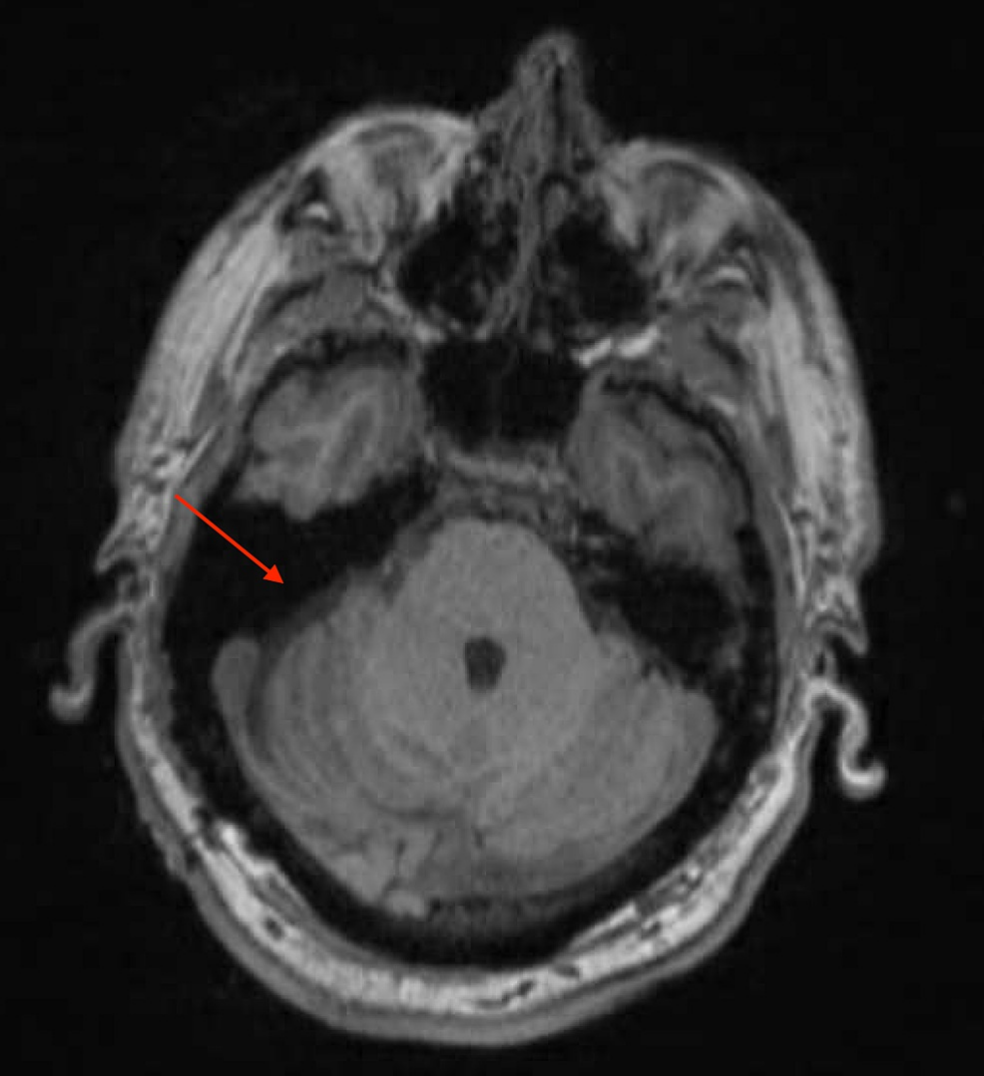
Postoperative T2-weighted MRI with contrast demonstrating postsurgical changes, with the site of resection indicated by the red arrow
